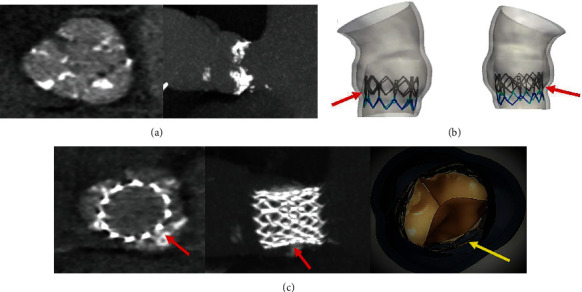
Biomodeling of SAPIEN XT starting from the CT scan. (a) CT scan showed calcifications of aortic valve and root; (b) modeling of the balloon-expandable system showing an incomplete deployment (red arrow); (c) thrombotic formation (red arrow) in correspondence with the distortion of the stent and reduced mobility of the leaflet of the bioprosthesis (yellow arrow).
